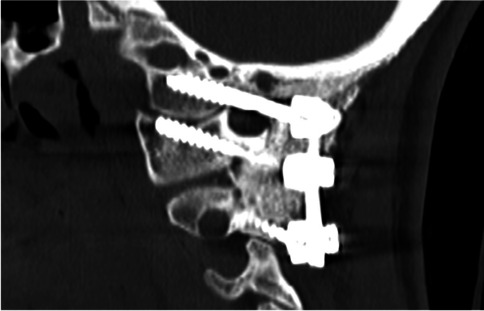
Sagittal 2D CT with C0-2 construct with condylar screws and mature fusion
